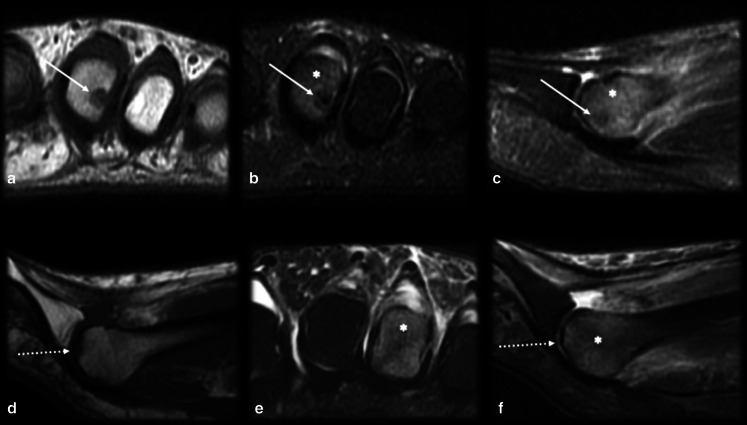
Osteoid osteoma versus subchondral fracture. (a–c) Female, 32 years old, presenting with metatarsalgia for 2 months. T1 coronal (a) and T2 FS coronal (b) and sagittal (c) MRI showed a mineralized nidus (arrows) with reactional bone marrow and adjacent edema (asterisk). (d–f) Female, 33 years old, presenting with metatarsalgia for 3 weeks. T1 sagittal (a) and T2 FS coronal (b) and sagittal (c) MRI showed a subchondral fracture (dotted arrows) with bone marrow edema (asterisk)
